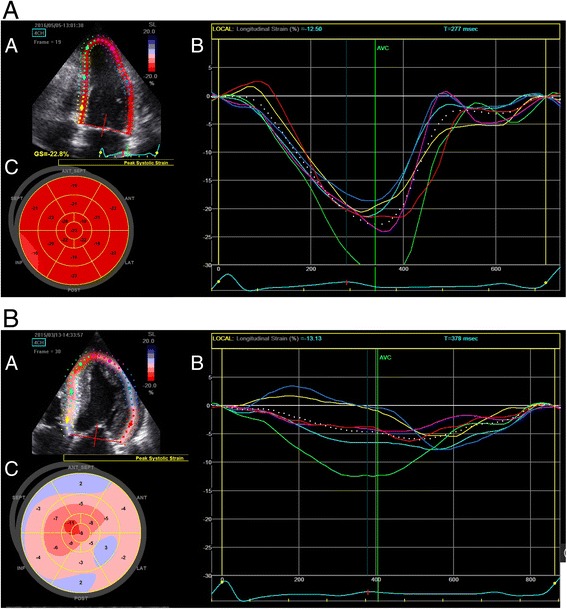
a Speckle-tracking analysis of a patient with normal systolic left ventricular (LV) function. 2D image showing speckles within the LV being tracked by the ultrasound Machine Software (*A*). Graphical representation of movement of speckles throughout the cardiac cycle (x-axis, longitudinal strain; y-axis, time in msec), with each *line* representing a different segment of the LV; large negative values represent movement of speckles towards one another during contraction representing normal function (*B*). Bullseye map showing global longitudinal strain values throughout the LV (*C*). b Speckle-tracking analysis of a patient with severely reduced left ventricular (LV) systolic function. A 2D image showing speckles within the LV being tracked by the ultrasound machine software (*A*). Graphical representation of movement of speckles throughout the cardiac cycle (x-axis, longitudinal strain; y-axis, time in msec) with each *line* representing a different segment of the LV; note smaller negative values with variable time to peak strain representing reduced LV function with mechanical dyssynchrony (*B*). Bullseye map showing global longitudinal strain values throughout the LV; *blue zones* represent areas of the LV where there is lengthening of the segments during systole rather than shortening (*C*)
